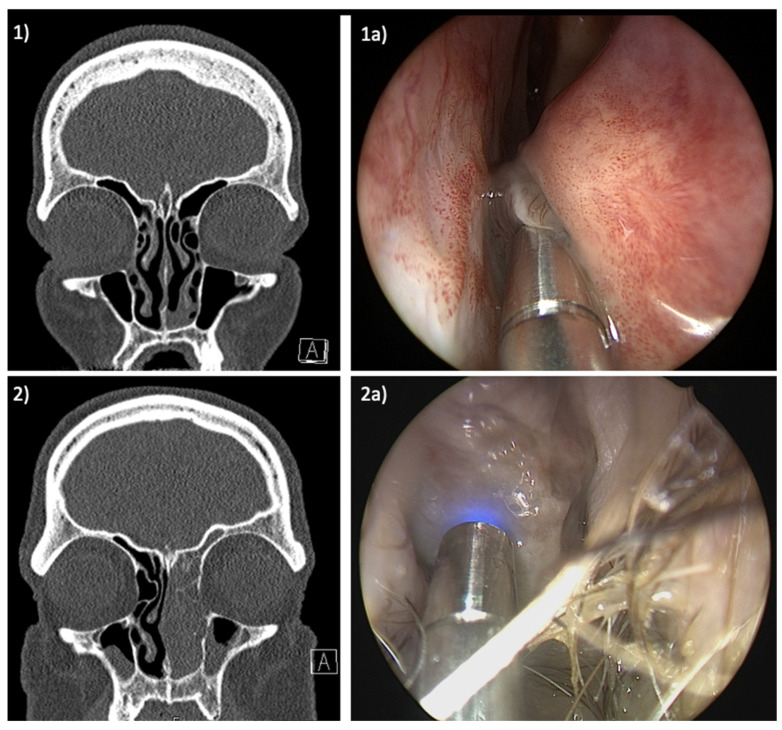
Radiologic and endoscopic findings: (1) CT-scan of patient I with SNMM of the floor of the left nasal cavity and the lower part of the inferior turbinate, (1a) endoscopic view of the laser probe applied on the tumor, (2) CT-scan of patient II with SNMM completely obstructing the left nasal cavity, (2a) endoscopic view of the laser probe applied on the tumor in the left vestibule.
